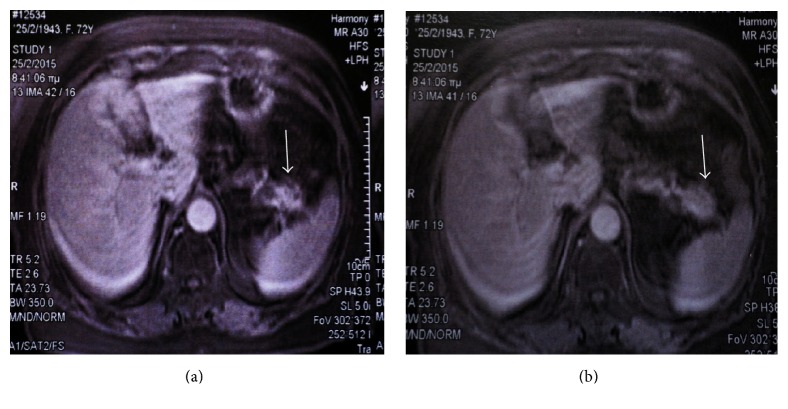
A mass in the tail of the pancreas with (a) strong enhancement and with (b) rapid washout, MRI images.
